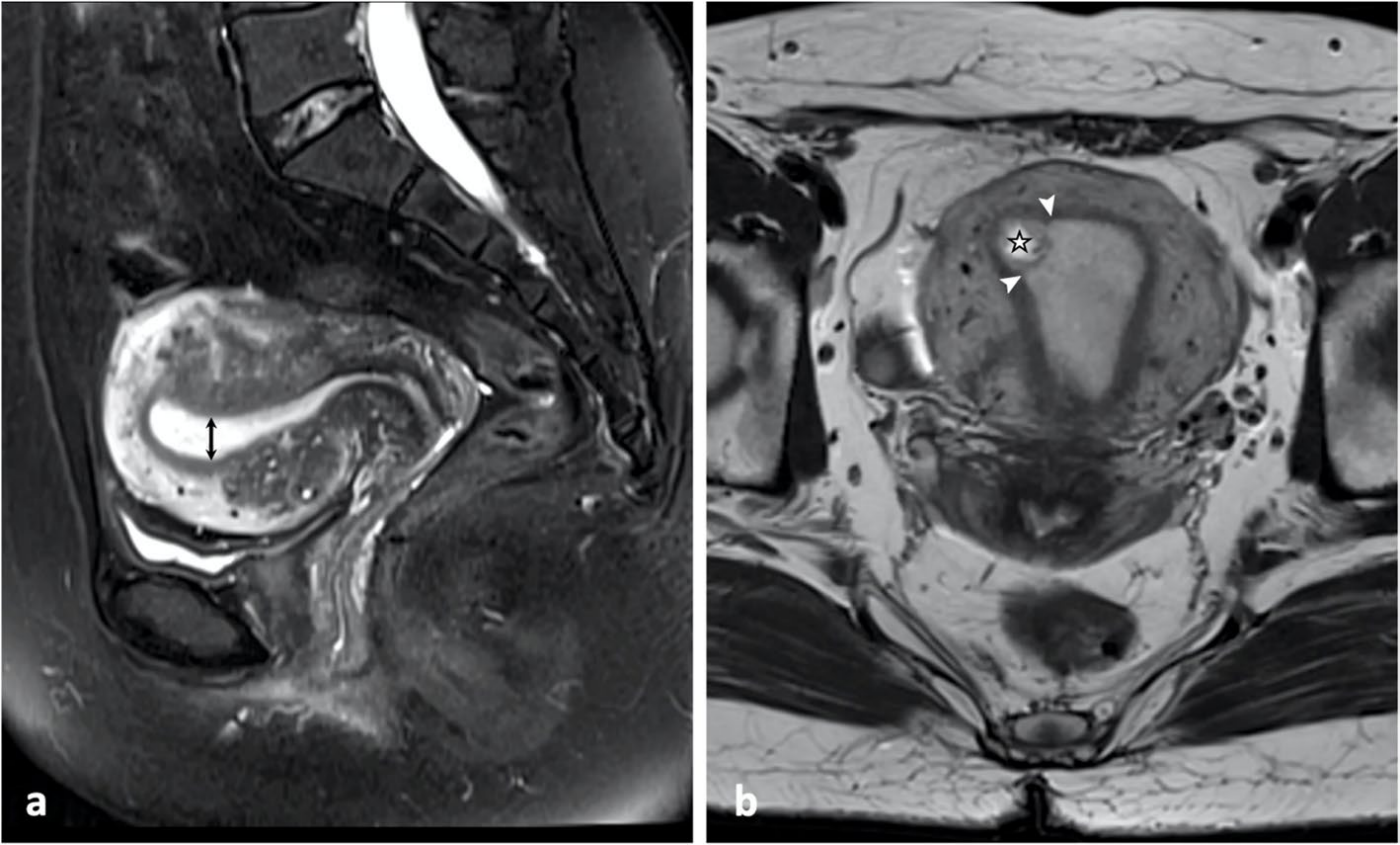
MRI in a 32-year-old patient with below-cutoff (11.5 mm) endometrial thickness. (a) Uterine sagittal fat-suppressed, T2-weighted imaging showed an endometrial thickness (double-ended arrow) of 8.6 mm. (b) Uterine coronal T2-weighted imaging showed a gestational sac (star) and interrupted peri-gestational sac endometrium (arrow heads)
